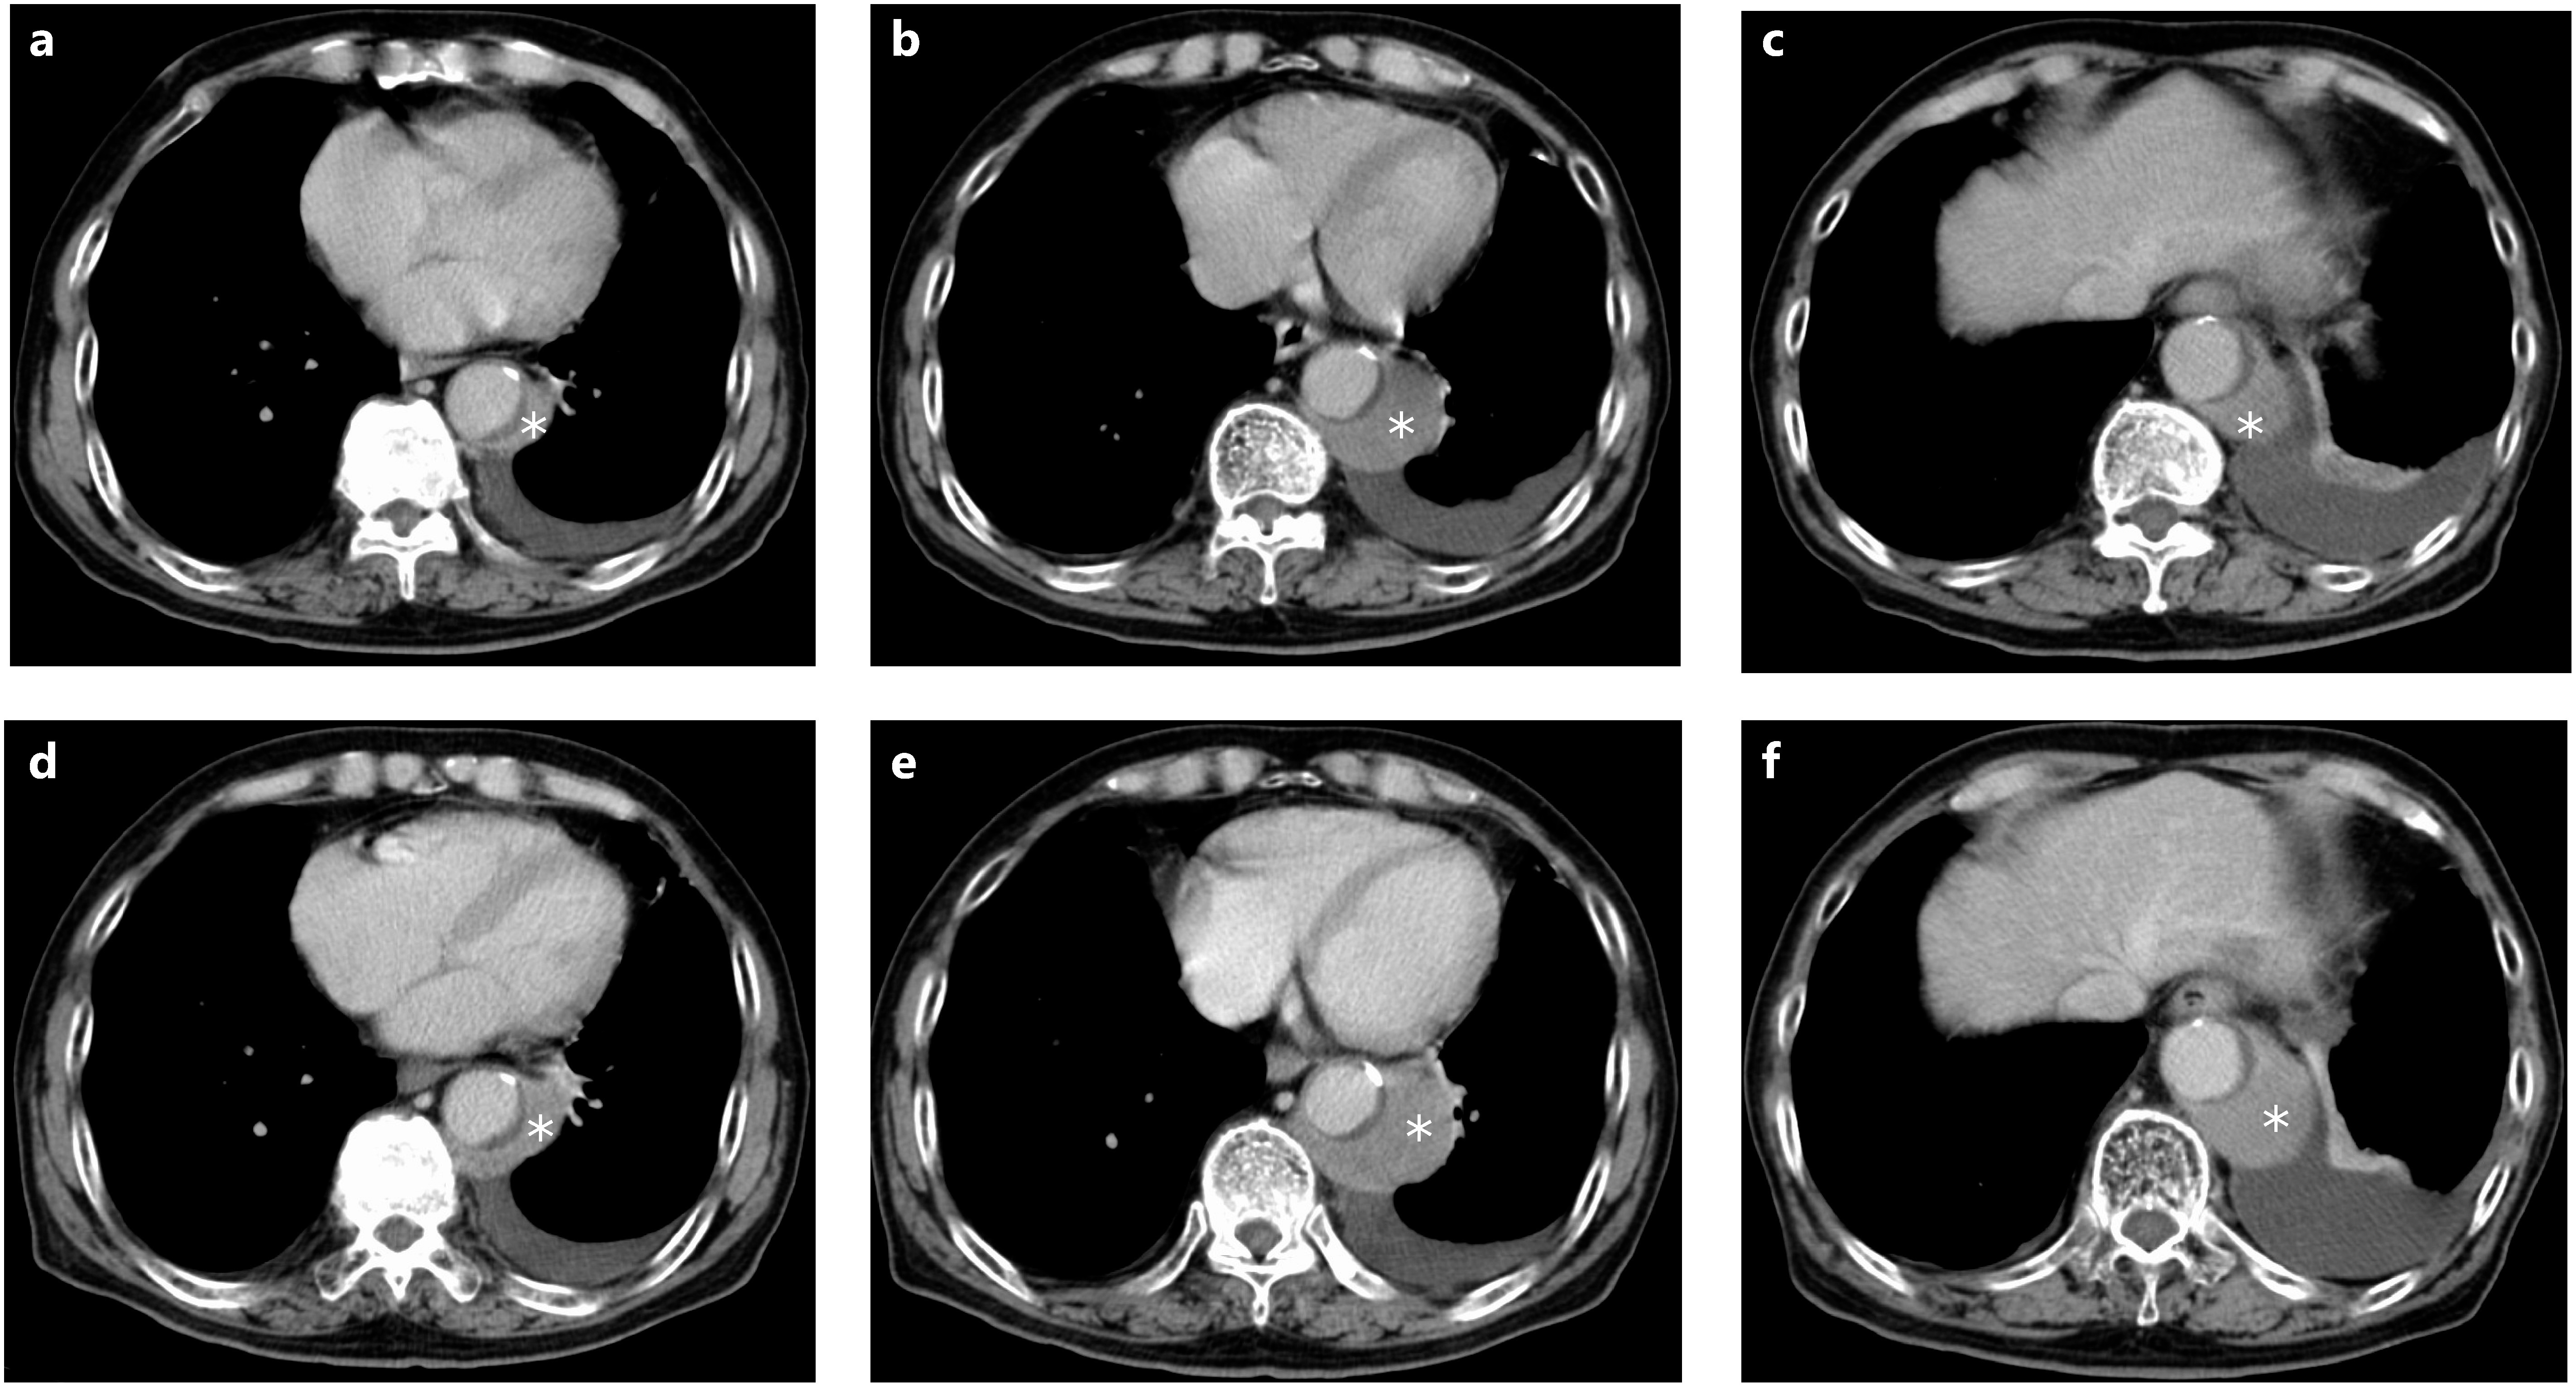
Fig. 1 Computed tomography (CT) images a–c. CE-CT on admission showed a false lumen-like structure. d–f. CE-CT showed rapid increase in the maximum diameter of the dissected aorta one month after onset. The asterisk (*) indicates a false lumen-like structure.
